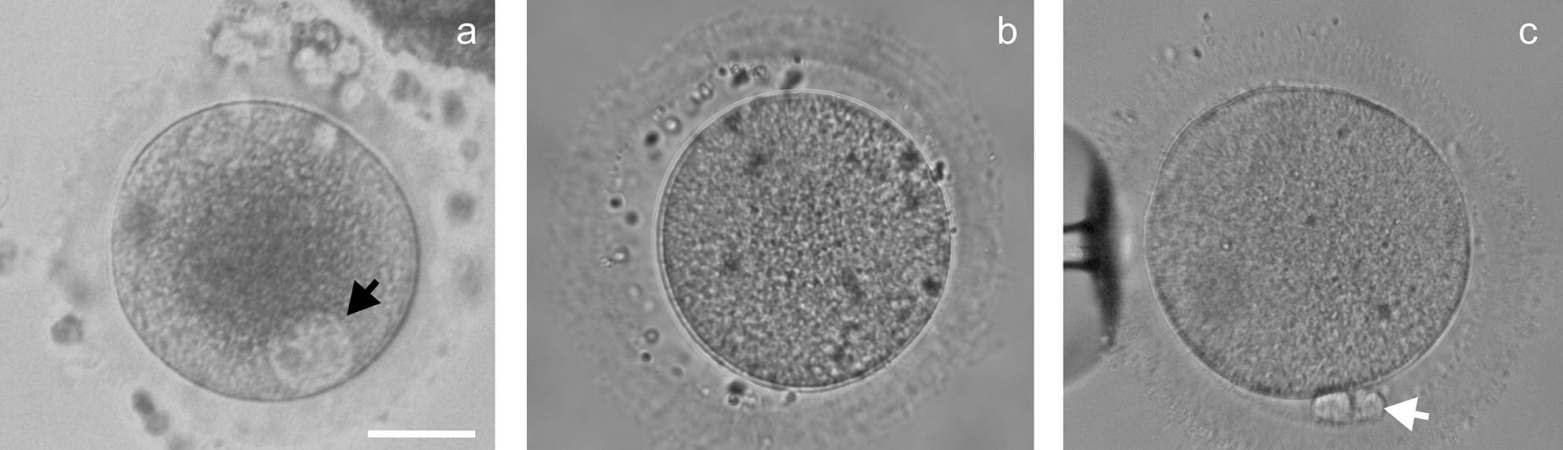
Oocytes after transvaginal sonographically controlled follicle punctures and cumulus removal. The images were taken during initial examination after punctures. GV (a) and MI (b) oocytes are excluded from the egg donation program. Oocytes at MII stage (c) are used in assisted reproductive technologies. Black arrow—the nucleus with a kariosphere, white arrow—a polar body. Scale bar: 50 μm.
